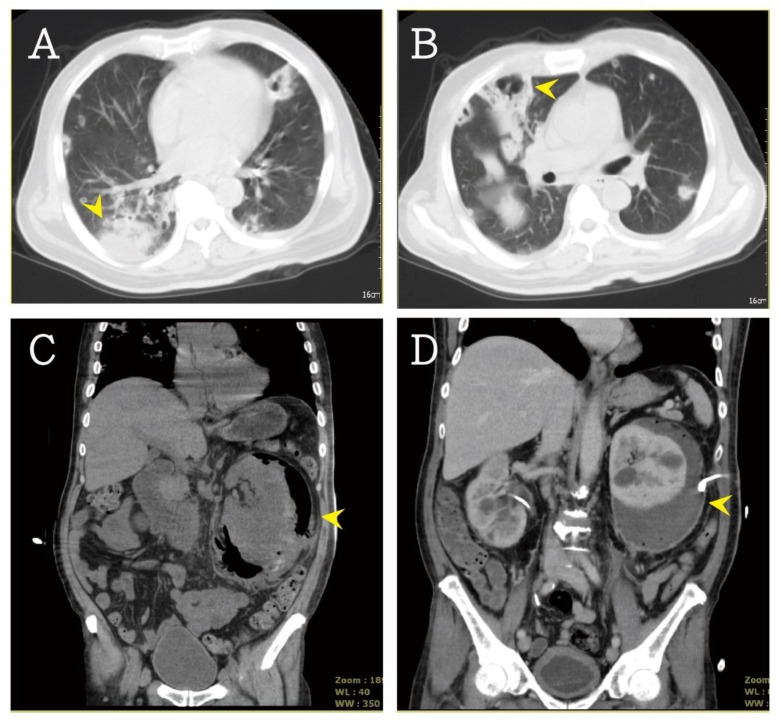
(A,B) Diffuse bilateral nodular densities with poor margin and cavitation was noted (arrow head) in CT scan. (C) An enlarged kidney, destroyed renal parenchyma with fluid and gas collections in renal capsule, was found (arrow head). (D) After emergency drainage (arrow head), the fluid accumulation was improved.
